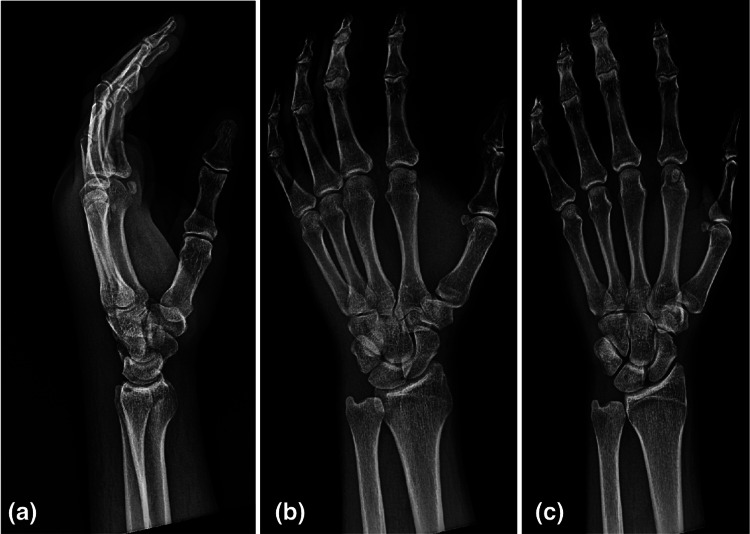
X-rays of the left hand with no osseous abnormalities or foreign bodies identified. (a) Notes a lateral X-ray of the left hand. (b) Oblique view of the left hand. (c) Posterior-anterior view of the left hand.
